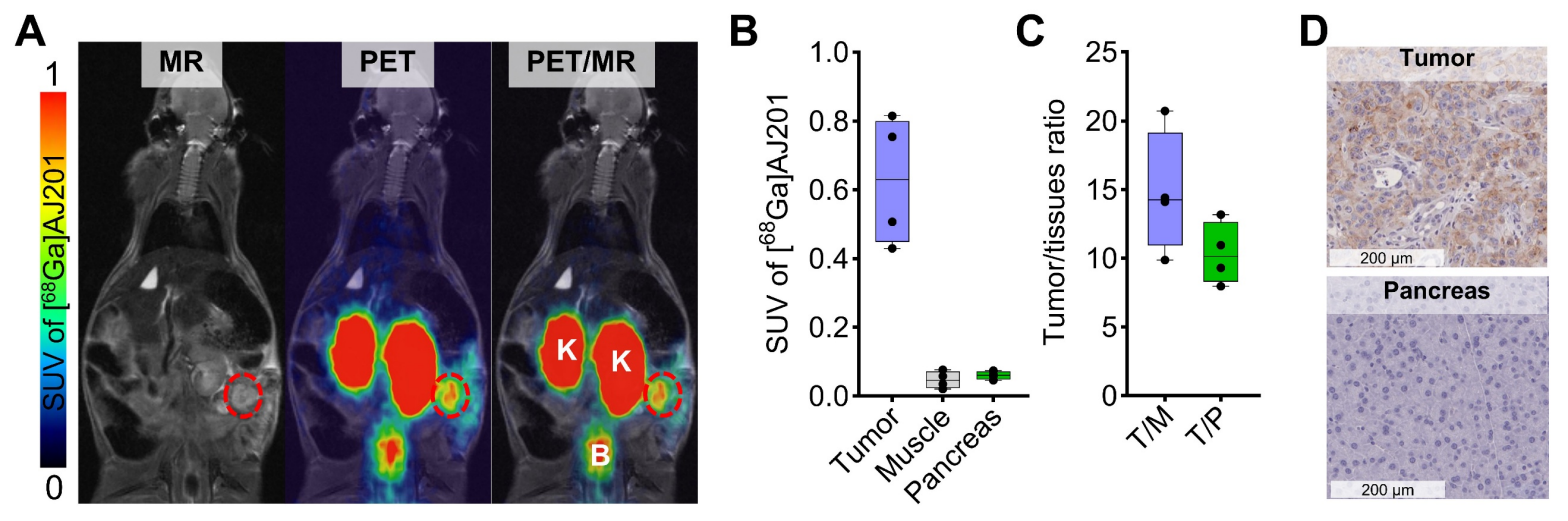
In vivo* distribution of [^68^Ga]AJ201 in Panc1-orthotopic tumor model. A) Coronal sections of the fused PET/MR images showing [^68^Ga]AJ201 distribution. Primary tumor is indicated by red circle; K = Kidney, B = Bladder. B) Quantification of accumulated activity in tumor, muscle and pancreas of images shown in panel A (n = 4-5) C) tumor-to-muscle (T/M) and tumor-to-pancreas (T/P) ratios from the images shown in panel A (n = 4) D) EphA2 IHC of orthotopic Panc1 tumor and normal pancreas to validate the EphA2 expression. Panc1 orthotopic tumor model was generated by surgically implanting a 3-5 mm³ section of Panc1 tumor xenograft directly onto the pancreas. The implanted tumors were allowed to grow for 10 days, after which they were ready for imaging. At this stage, MRI measurements indicated that tumor sizes ranged from 10-50 mm^3^. data in figure B and C is shown as box and whisker plots (median ± IQR) showing all data points (n = 3-5).
